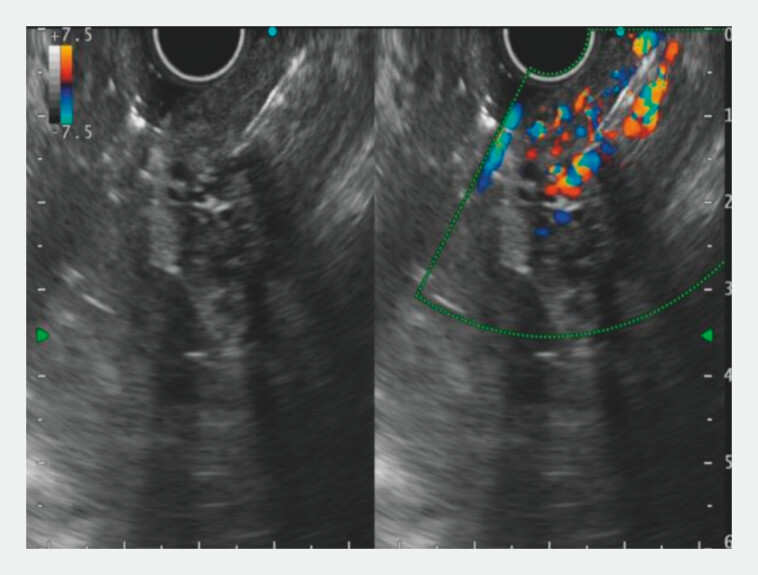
Endoscopic ultrasonography (EUS) with color Doppler evaluation of blood flow in gastric varices and EUS-guided injection of cyanoacrylate.
